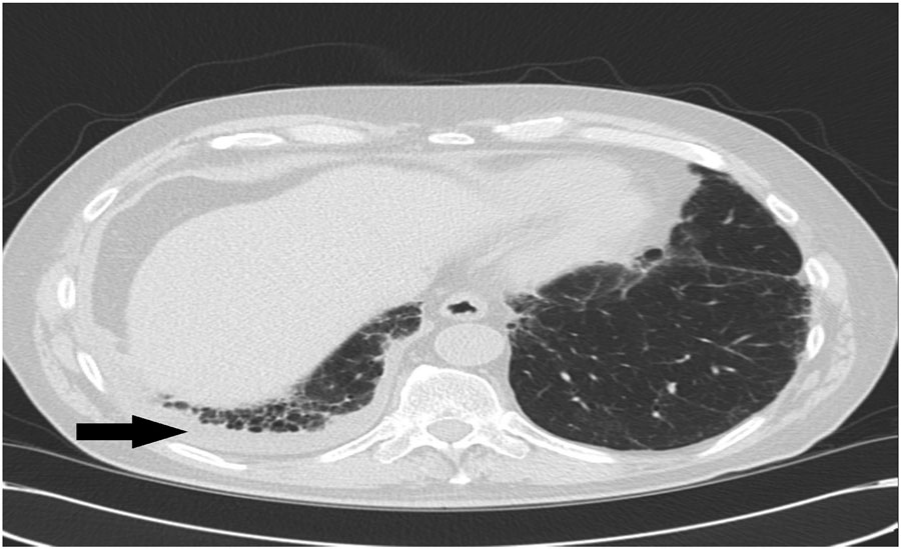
High resolution CT chest with small right pleural effusion (Black arrow). CT, computed tomography
